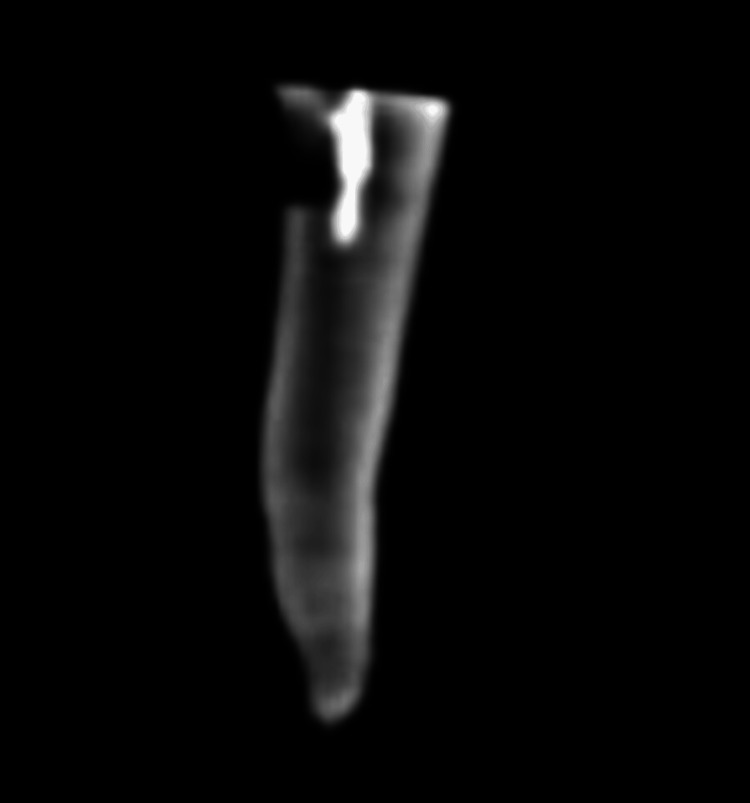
CBCT image after using the Neoendo retreatment files and obturating with the AH Plus sealer The white discontinuous shade (radio-opacity) at the center of the tooth shows the remnant of the AH Plus sealer after retreatment with the Neoendo files. CBCT: cone-beam computed tomography
